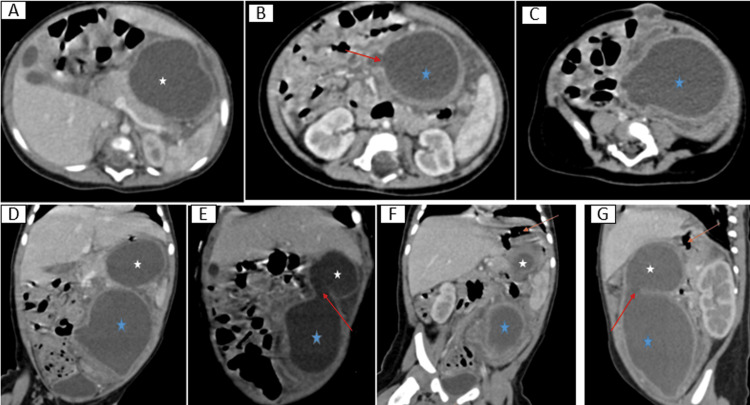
Contrast-enhanced CT images in axial (A, B, C), coronal (D, E, F), and sagittal (G) views of a four-month-old infant with a bilobed GDC. The images demonstrate a bilobed intraperitoneal cystic mass extending from the left hypochondrium to the left iliac fossa, measuring 51 mm × 47 mm × 117 mm (D, E, G). The bilobed structure exhibits clear communication between the two lobes (E, F) but no connection with the digestive lumen. White star (★): primary GDC. Blue star (★): intra-epiploic extension of the GDC. Red arrows (→): communication between the two lobes (B, E, G). Orange arrows (→): areas of close adhesion to the gastric wall (F, G). GDC, gastric duplication cyst
